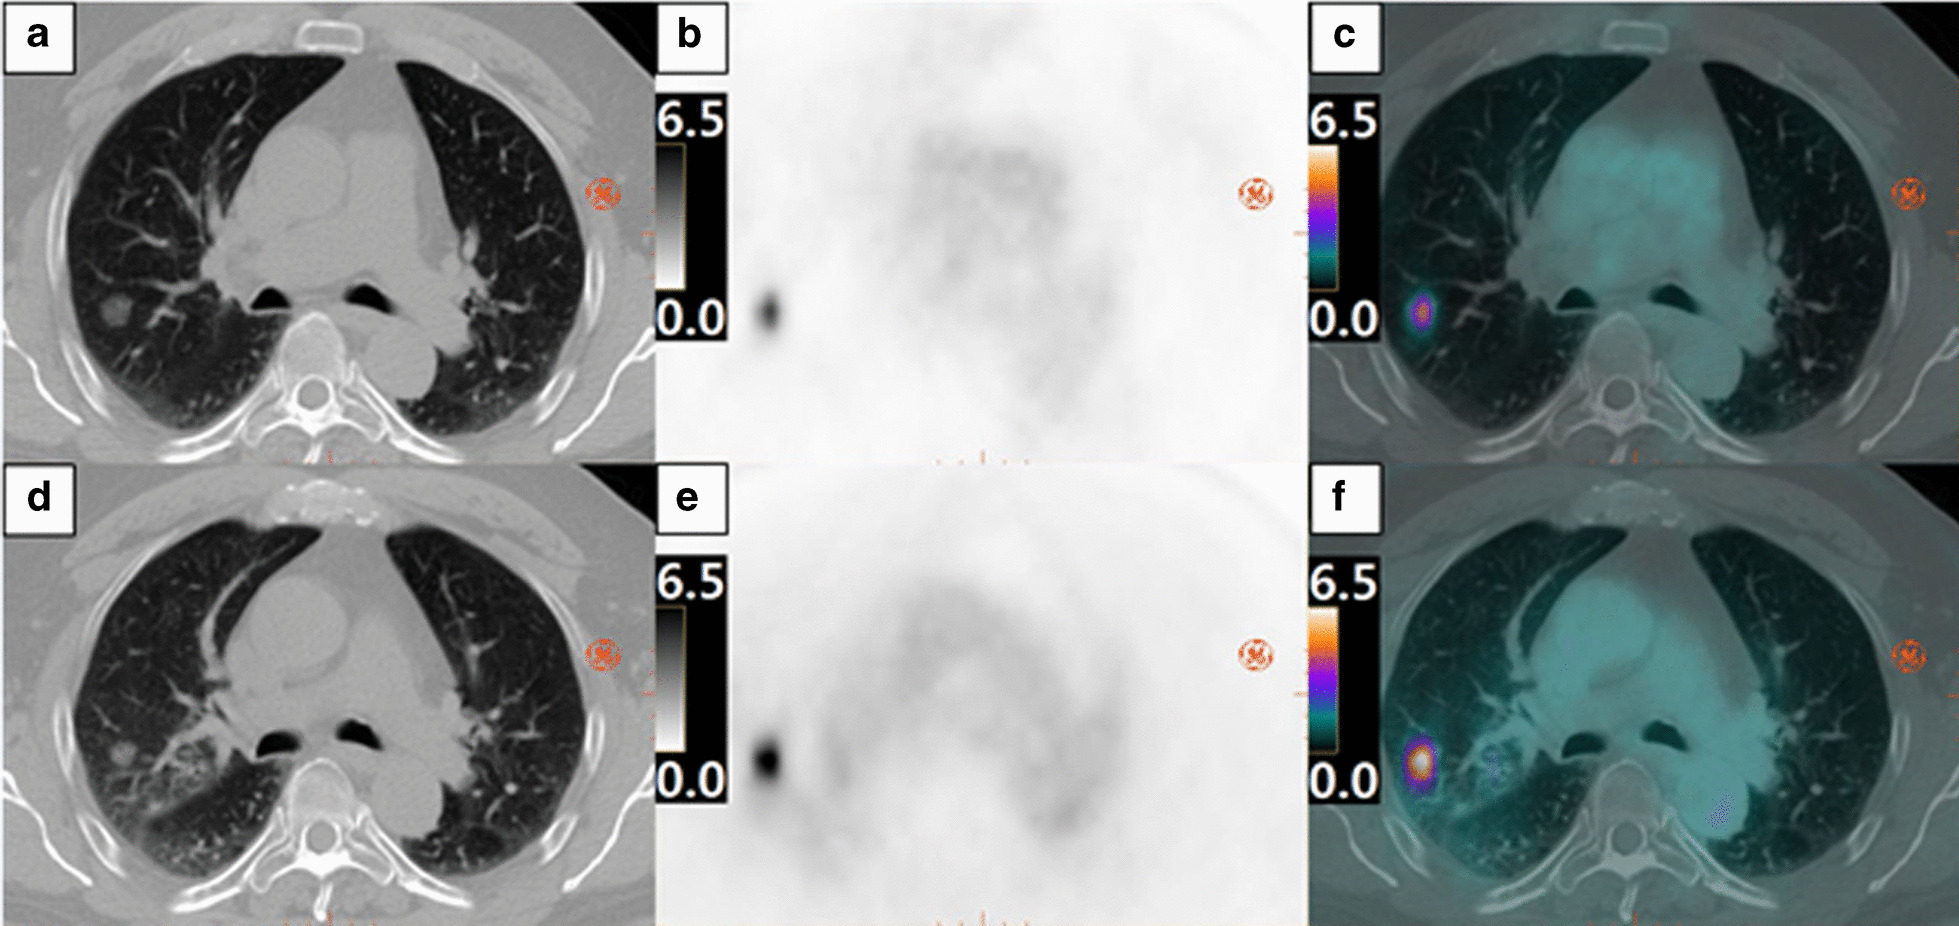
Example of PERCIST failure. Pre-SABR images of pCR patients on CT, PET SUV (range 0–6.5 g/mL), and PET/CT fused are shown in a, b and c, respectively. Post-SABR CT, PET and PET/CT fused images are shown in d, e and f, respectively. *PERCIST* PET Response Criteria in Solid Tumours, *SABR* stereotactic ablative radiation therapy, *pCR* pathologic complete response, *CT* computed tomography, *PET* positron emission tomography, *SUV* standardized uptake value
